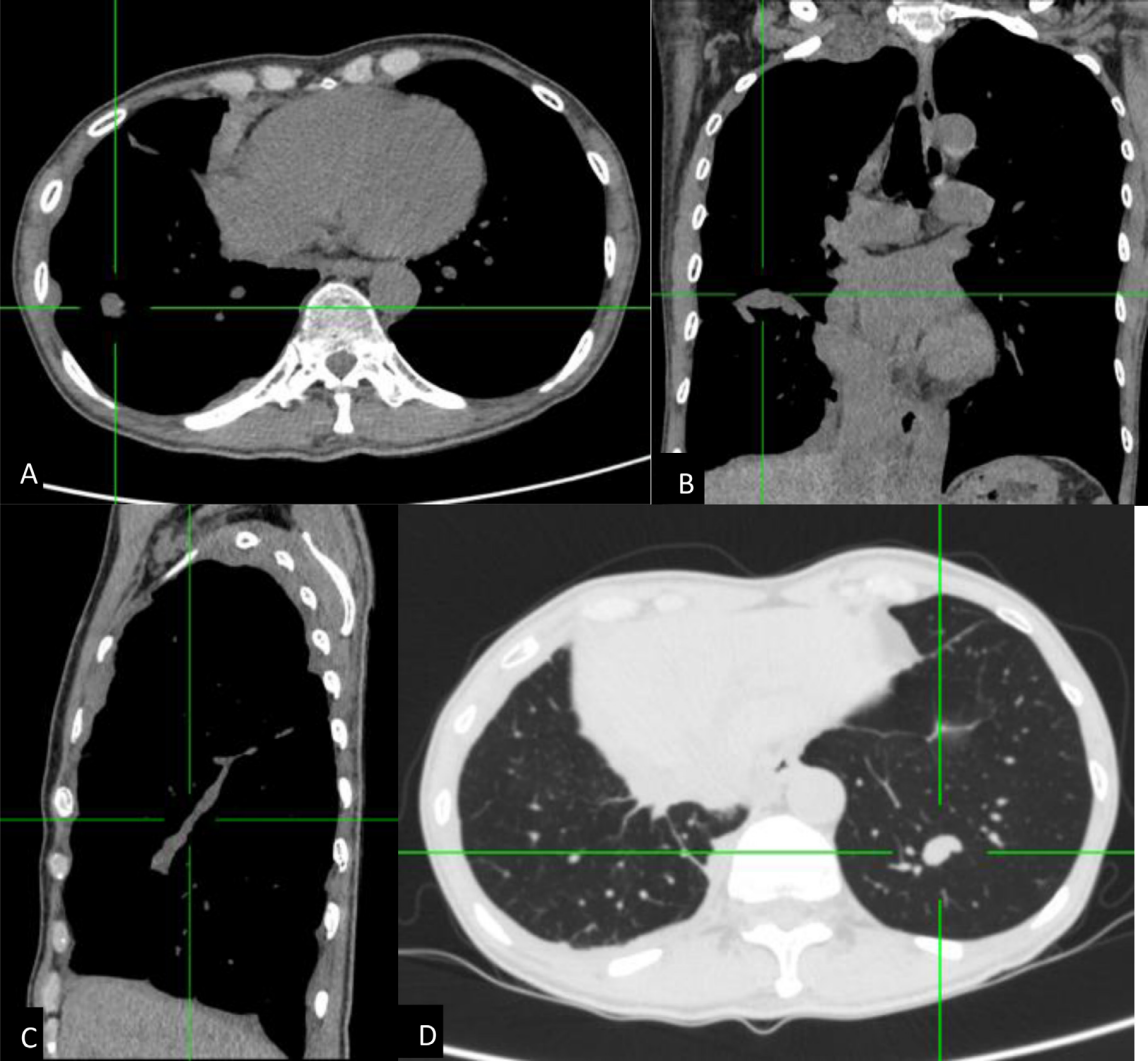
Post-treatment unenhanced chest CT scan in axial (A) with coronal- (B) and sagittal-reformatted (C) plane show marked improvement in overall pleural and fissure thickening. Multiple right lung nodules can be well appreciated with the right lung fully expanded and without right pleural effusion. There is also a reduction in the greatest dimension of the left lung nodule (D), measured at approximately 1.1 cm (greater than 20% reduction).
